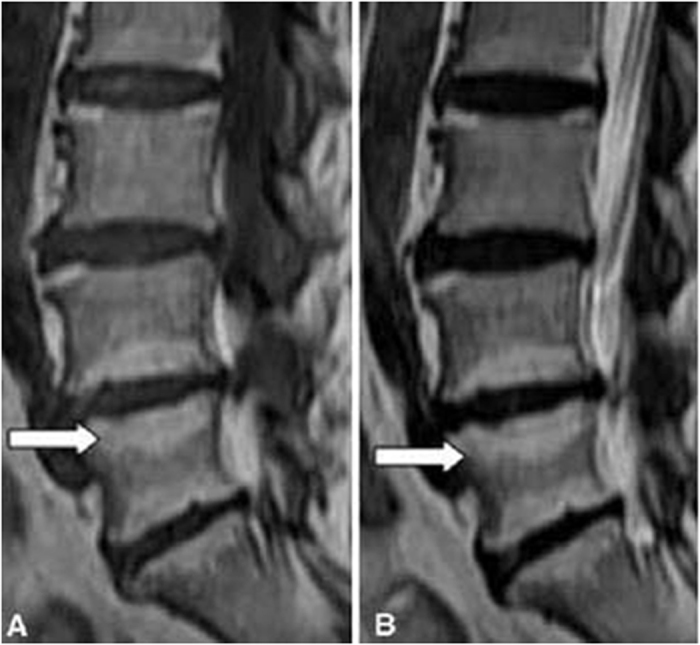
Magnetic resonance images of type II MCs at the L4–5 and L5-S1 levels in the lumbar spine.
